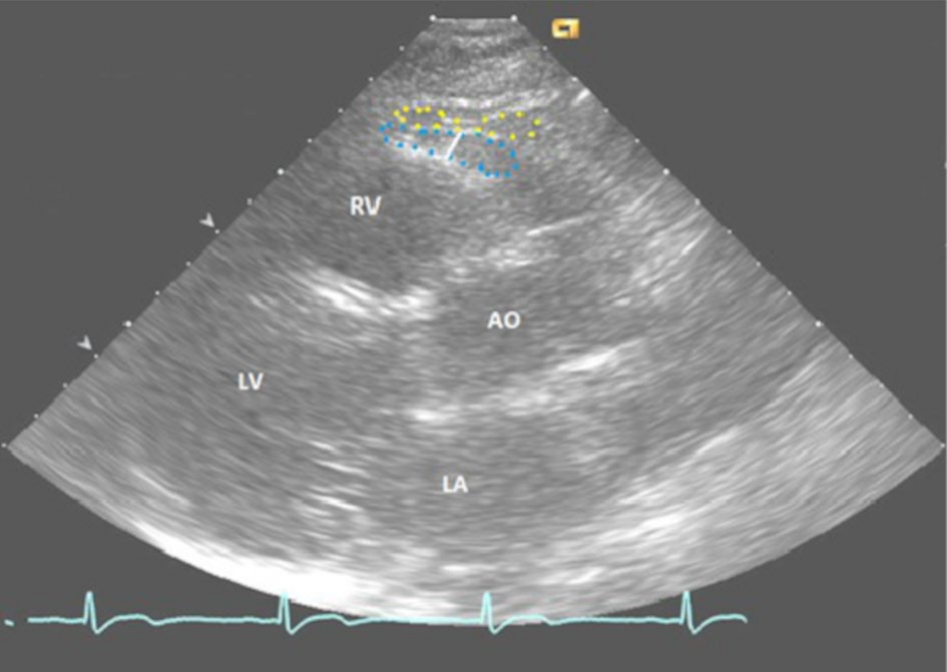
Example image of echocardiograms with visible pericardial and epicardial layer. Epicardial fat depot (blue dots). Pericardial fat depot (yellow dots). EAT thickness measured perpendicular to right ventricle at the level of aortic annulus (white line). *RV* right ventricle, *LV* left ventricle, *LA* left atrium, *AO* aorta.
